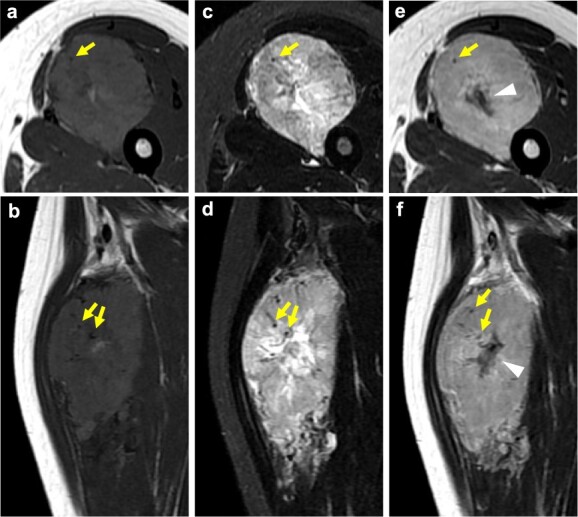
Radiological features of alveolar soft part sarcoma. Magnetic resonance (MR) images of a tumour involving the quadriceps femoris in a 19-year-old female. (a, b) Axial (a) and sagittal (b) T1-weighted MR images. The tumour signal intensity is higher than the muscle signal intensity. Peritumoral abnormal vessels (arrows) can be seen in the tumour. (c, d) Axial (c) and sagittal (d) T2-weighted MR images. The tumour shows high signal intensity, which was not affected by fat suppression. Peritumoral abnormal vessels (arrows) can be seen. (e, f) Axial (e) and sagittal (f) gadolinium-enhanced T1-weighted MR images. The tumour shows a central area without enhancement corresponding to the necrotic area (marks). Peritumoral abnormal vessels (arrows) can be seen.
